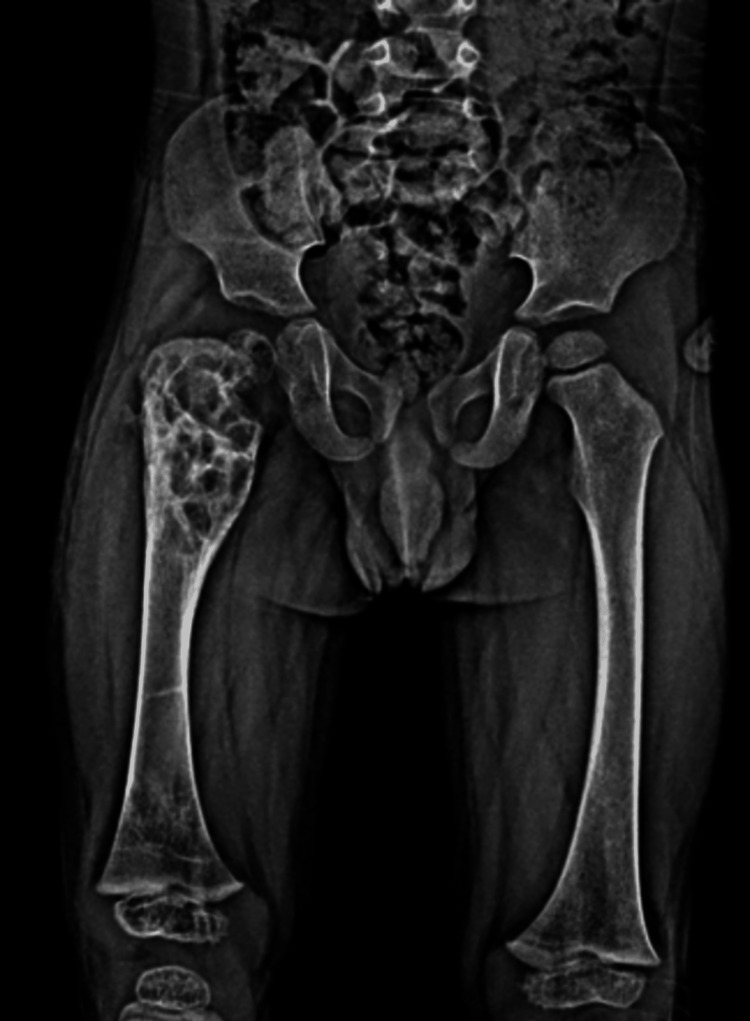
Sixth-month control radiograph of a clinically asymptomatic patient
